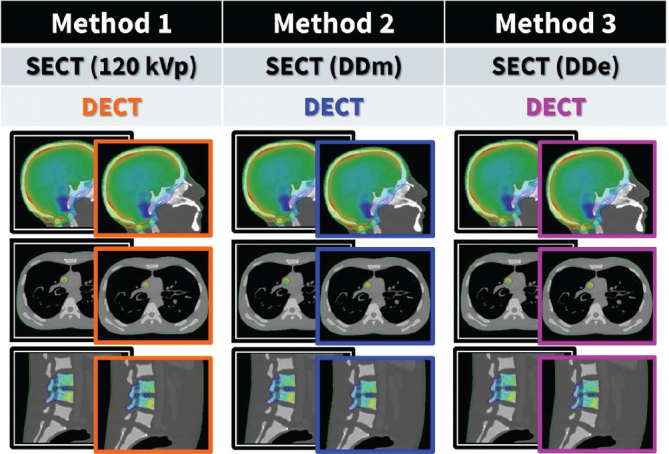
In three methods the correspondence of matched pairs of single-energy computed tomography (SECT) and dual-energy computed tomography (DECT) were tested in relation to dose calculation in three anatomical regions (head, thorax, abdomen/pelvis).
